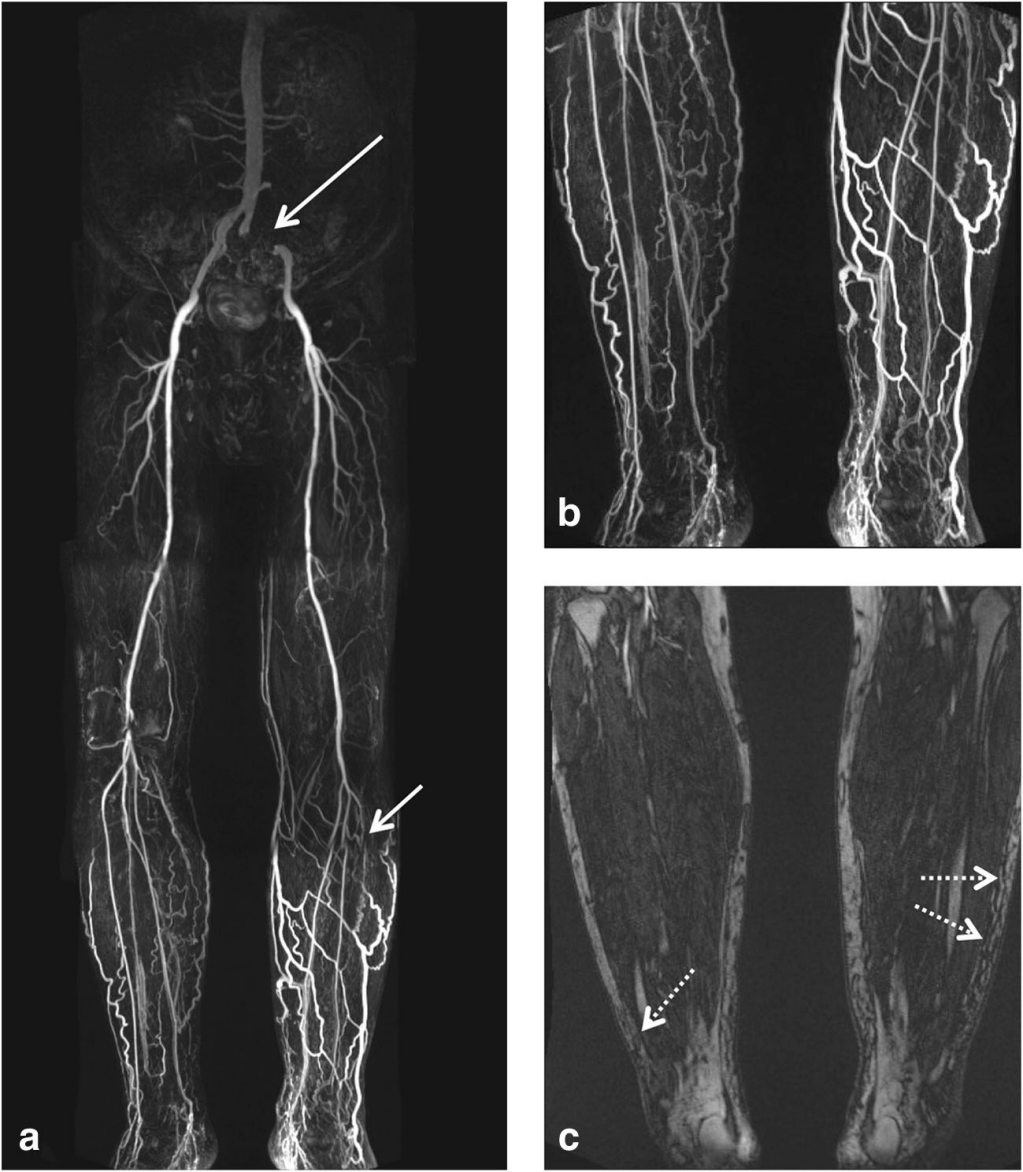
Run-off MR angiography in a 66-year-old male patient with known stage IV peripheral artery disease. Subtracted images post contrast show occlusion of the left common and internal iliac arteries (long arrow in a) and occlusion of the left anterior tibial artery (short arrow in a). Substantial varicosis of the superficial veins of both lower legs is also noted (magnified in b). T1-weighted pre-contrast image shows subcutaneous lower leg oedema (dotted arrows in c)
